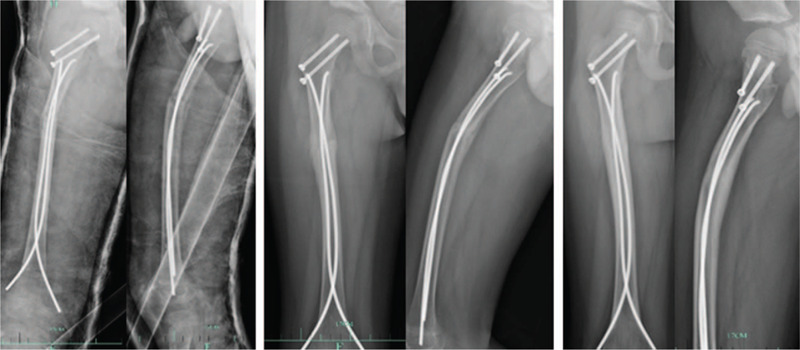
The above picture shows the X-ray findings at 1 month, 3 months, and 7 months after surgery. The osteophyte growth was observed in 1 month and the bone healing was performed in 3 months.
